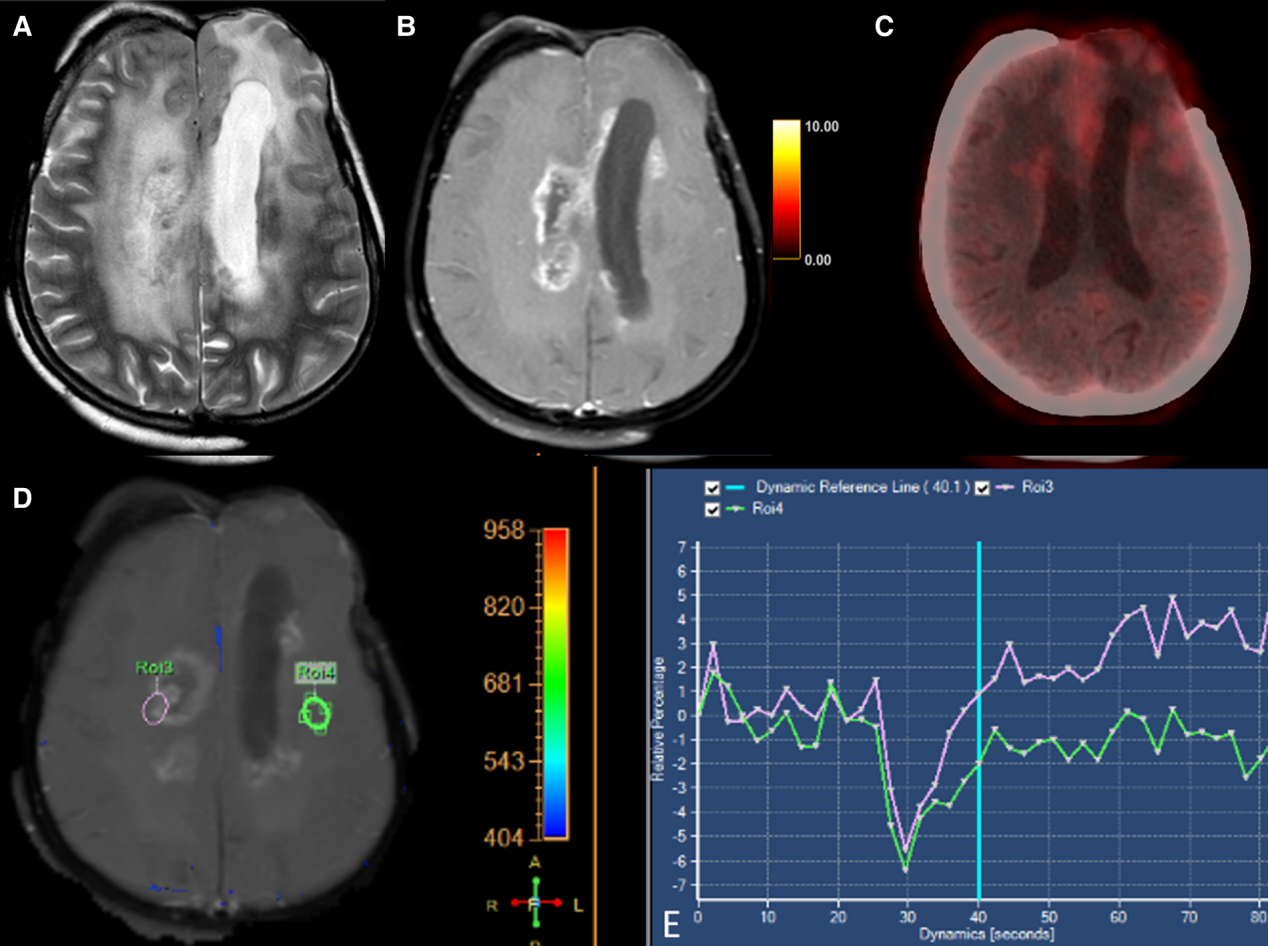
Axial T2-weighted (A), T1 + Gd (B), perfusion curve (D,E) and FET-PET (C) images in a case of grade III oligodendroglioma shows T2 hyperintense areas in the right centrum semiovale with surrounding edema. This lesion shows a “Swiss-cheese” pattern of enhancement (B) and hypoperfusion (D). FET-PET (C) shows no significant uptake (T/W ratio: 1.6) in the same area. These features were suggestive of radionecrosis.
